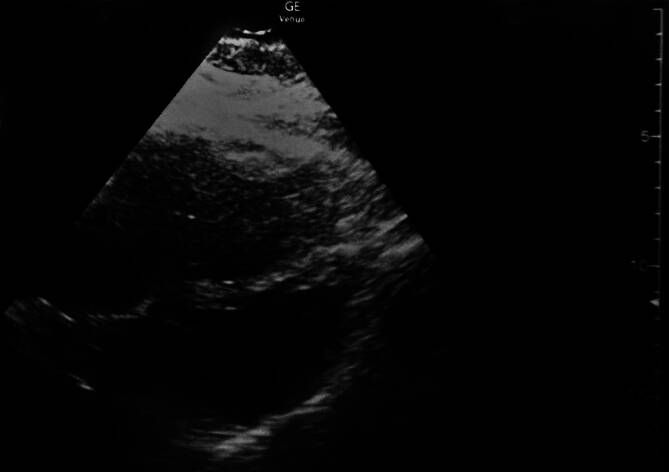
Notfallsonographiebefund mit „D sign“ des rechten Ventrikels
